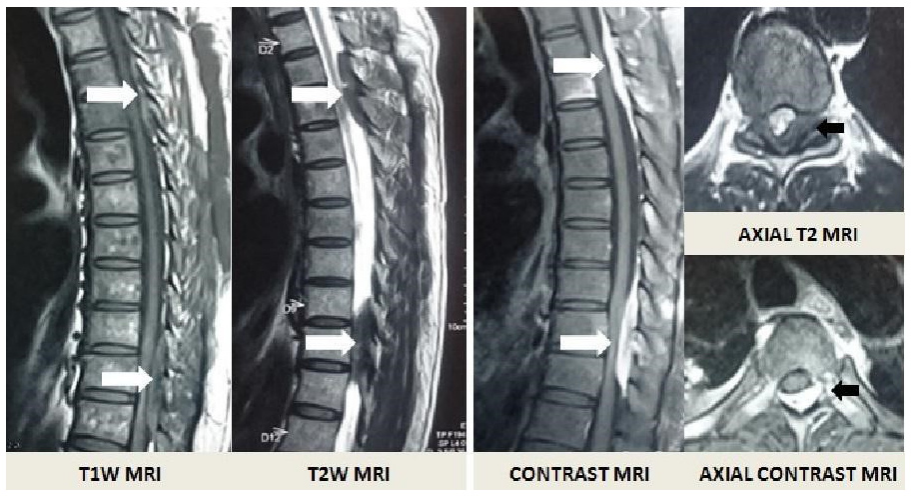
MRI images revealing the presence of multiple extradural enhancing lesions in the thoracic region.
